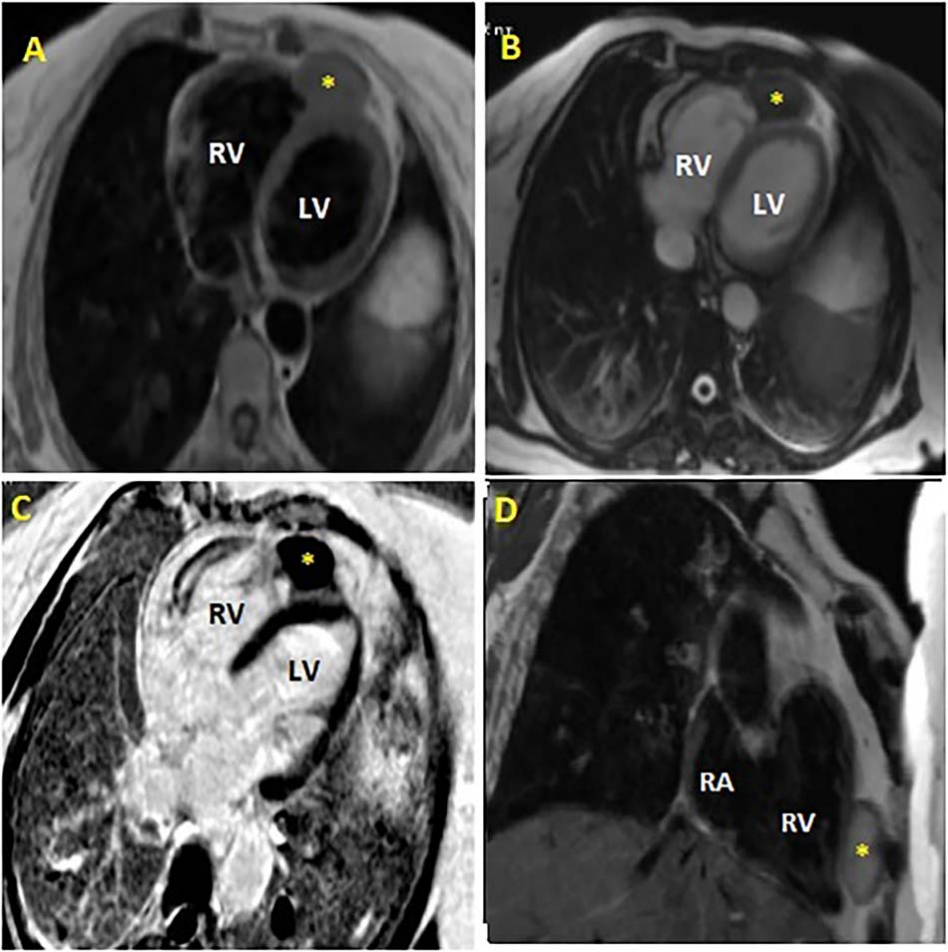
Cardiac magnetic resonance imaging (MRI) showing hydatic intrapericardial cyst (yellow scar) involving the myocardium at the right ventricular apex, with intermediate signal on T2-weighted imaging (A: axial view, D: sagittal view), iso-intense to myocardium on T1-weighted imaging (B: axial view) and hypo-intense to myocardium on late gadolinium enhancement (LGE) axial view (C). RA: right atrium; RV: right ventricle; LV: left ventricle.
